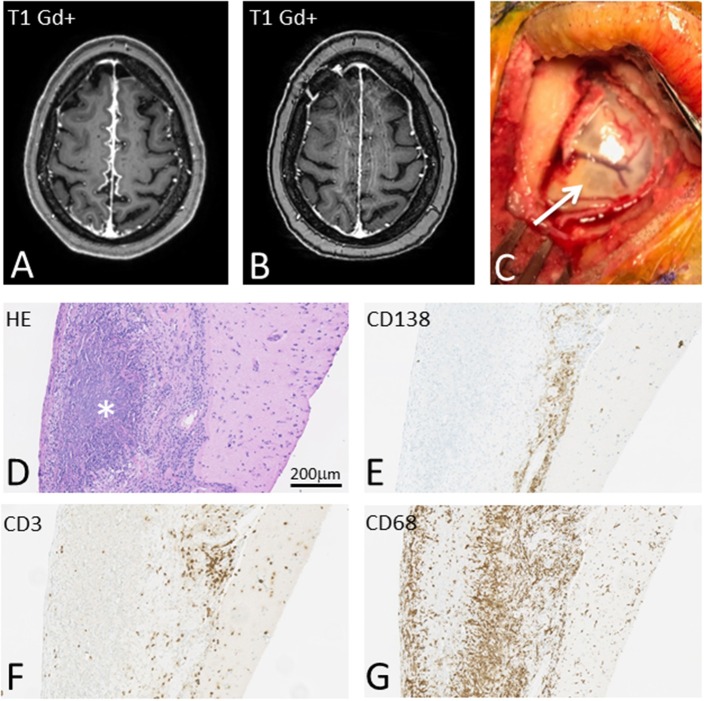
T1-weighted brain MRI showing interhemispheric leptomeningeal Gd+ enhancement before (A) and after (B) treatment with high dose steroids, Methotrexate and Rituximab. On gross inspection meninges appear severely inflamed (C) and pathological examination reveals massive meningeal granulomatous inflammation (D) with pre-dominant CD138 positive plasma cells (E), but also CD3 positive T cells (F). Massive infiltration with CD68 positive histiocytes with rheumatic granuloma formation was also seen (G).
